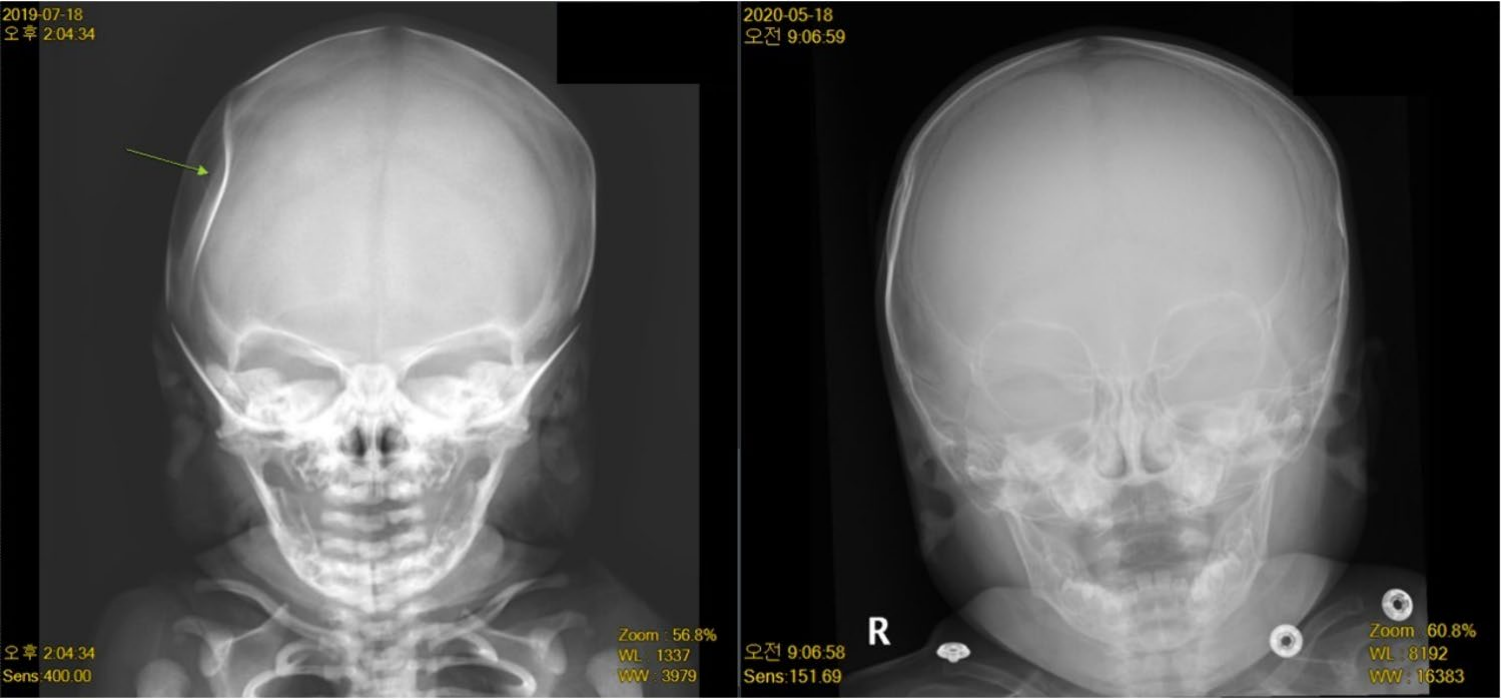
The skull X-ray images at the birth and at the latest visit of case 7 (left, depression fracture at the right parietal bone taken on day 1 after birth—July 18, 2019; right, interval improved state of right parietal area depressed fracture taken on when she became 10 months old—May 18, 2020). She was born through spontaneous vaginal delivery at gestational age of 39+0 weeks and her birthweight was 2,920 g (10–50 percentile)
